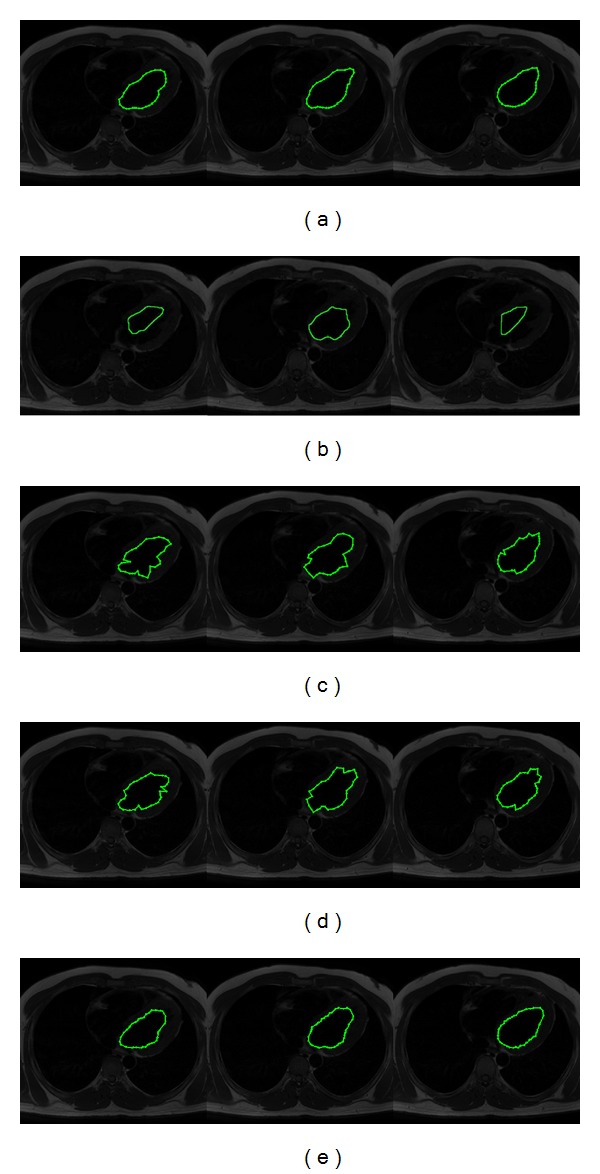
MR images (left ventricle segmentation): (a) manual delineation by experts, (b) results of Graph Cut method, (c) results of traditional ACM, (d) results of interactive Tseng method, and (e) results of proposed implementation.
